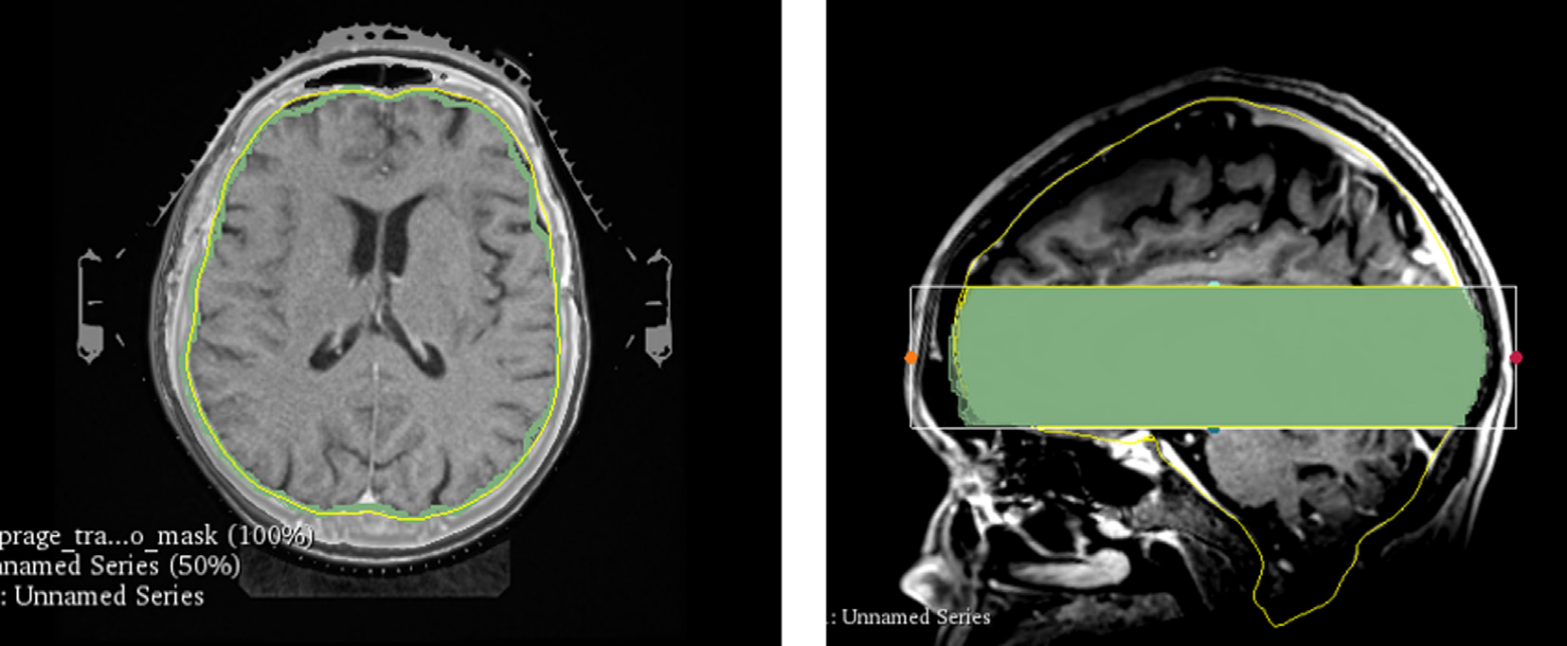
(a) A transversal view of the segmented brain of CT (yellow) and MRI (green), and (b) a sagittal view of the cropped brain segmentation used for segmentation-based evaluation. (For interpretation of the references to colour in this figure legend, the reader is referred to the web version of this article.)
